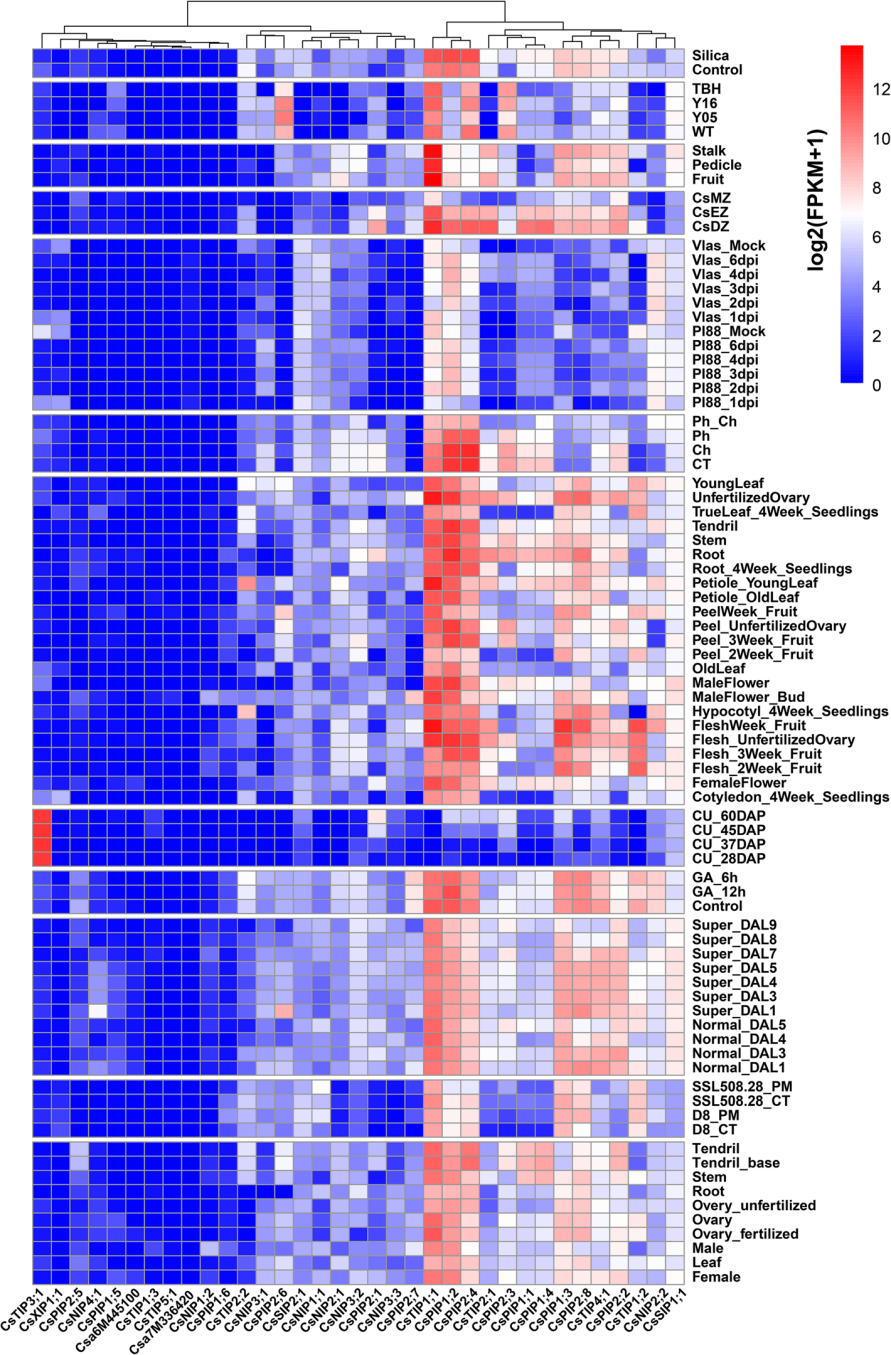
Expression pattern profiling of cucumber AQP genes. The different tissues used for expression analysis including root, leaf, seed, ovary, flower, fruit, pedicle, and stem. Experimental design: CT, control; DAP, day after pollination; DAL, the normal ovary blooms at 4–5 days after labeling (when the ovary is visible); tbh, a tiny branched hair mutant; Y05, fruit spine on fruits of 0.5 cm long; Y16, fruit spine on fruits of 1.6 cm long; WT, wild type cucumber; CsDZ, Root differentiation zone; CsEZ, Root elongation zone; CsMZ, Root meristematic zone; Ch, treated with chrysophanol; Ph, treated with physcion; GA_12h, treated with GA at 12 h; GA_6h, treated with GA at 6 h. Red indicates high concentrations, whereas low relative concentrations are deep blue. Data were normalized. Expression values are given after logarithmic transformation of RPKM (reads per kilobase of exon model per million mapped reads). All detailed information can be found in Cucurbit Genomics Database (http://cucurbitgenomics.org/organism/2)
